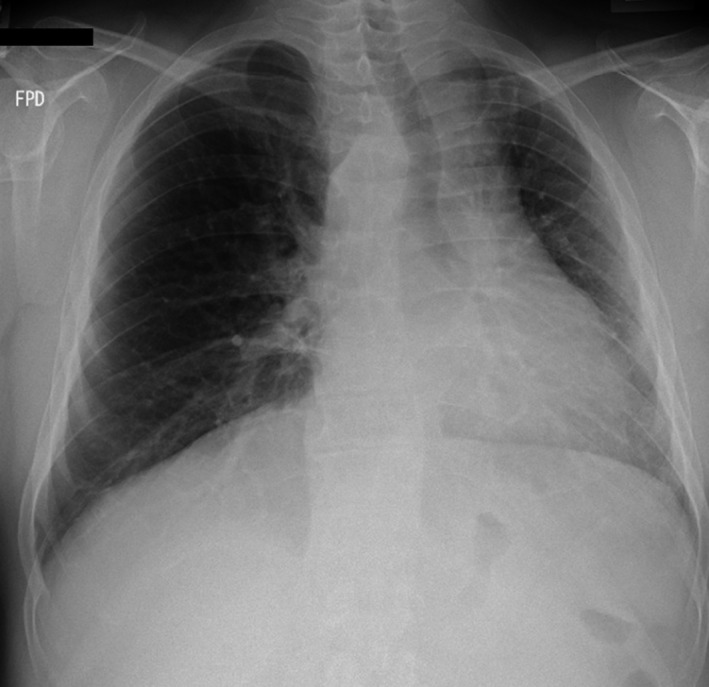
Preoperative images. Chest roentgenogram showed a mediastinal shift
